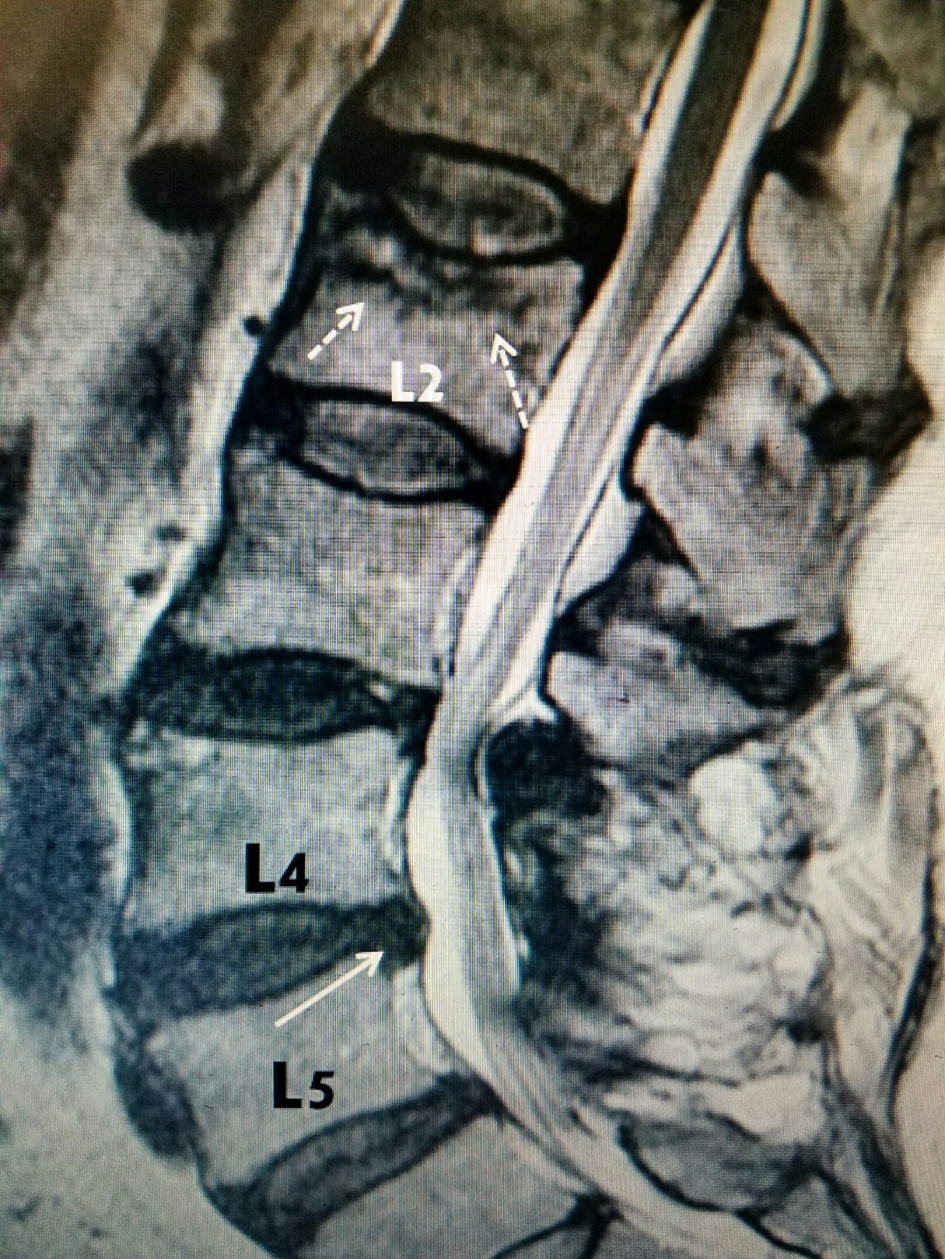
Lumbar spine MRI sagittal view in a patient with lower back pain demonstrating an acute L2 superior endplate fracture into the trabecular bone with edematous changes (dashed arrows) plus a small L4-L5 disc herniation (solid white arrow) with posterior facet hypertrophy at L3-L4
